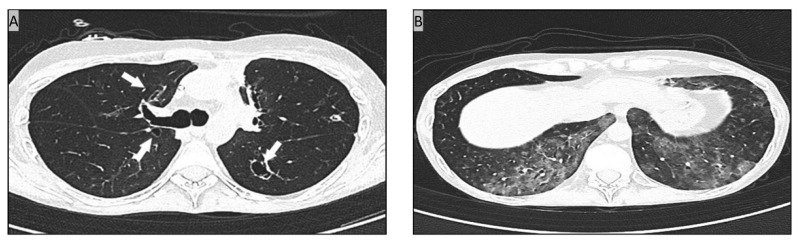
Chest HRCT showing pulmonary cavitated lesions with thick walls (arrows) and nodules in the upper lobes (A), and extensive ground-glass opacities in the lower lobes (B).
